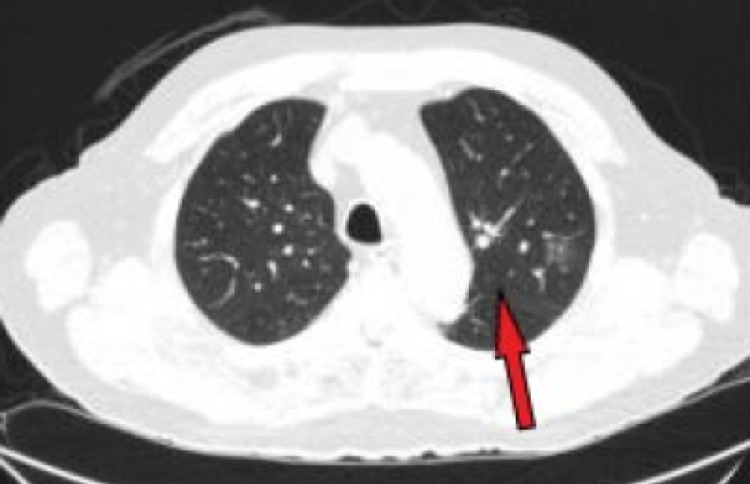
Postoperative CT demonstrating lung metastases. Non-contrast CT, axial views with two left lung hyperdensities, representing metastatic nodules (red arrow). CT: computerized tomography
